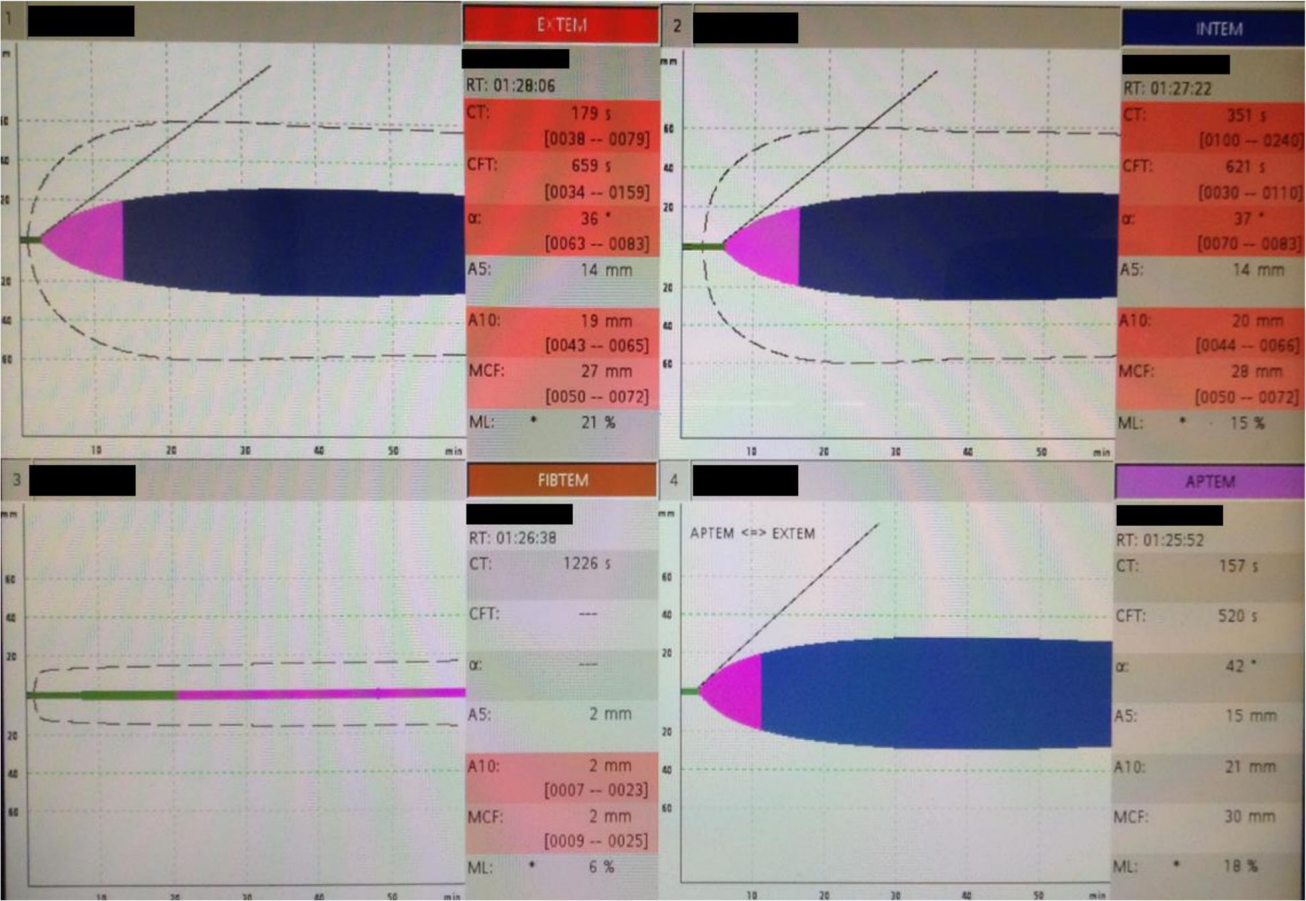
Results using the ROTEM system in a coagulopathic patient with complicated medical conditions. This was a ROTEM result in an 80-year-old woman who complained of vertigo. She had undergone artificial blood vessel replacement surgery for thoracoabdominal aortic aneurysm 8 years previously, and she had chronic hepatitis C, liver cirrhosis (Child-Pugh class B), and chronic atrial fibrillation. The ROTEM test revealed prolonged CT, prolonged CFT, low alpha angle, and low clot amplitude in every test in EXTEM and INTEM. Additionally, significantly reduced clot firmness in FIBTEM indicated fibrinogen dysfunction. This patient was not diagnosed with any acute cerebrovascular disease, and she was discharged on the same day
